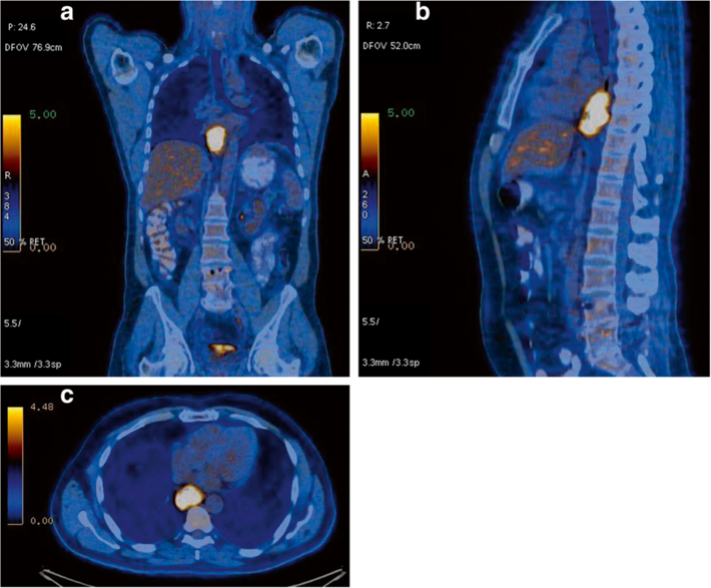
PET-CT demonstrates FDG uptake only in the esophageal lesion, with no evidence of lymph node or distant metastatic disease.a) front view; b) lateral view; c) PET-CT transversal section showing the esophageal tumor uptake.
